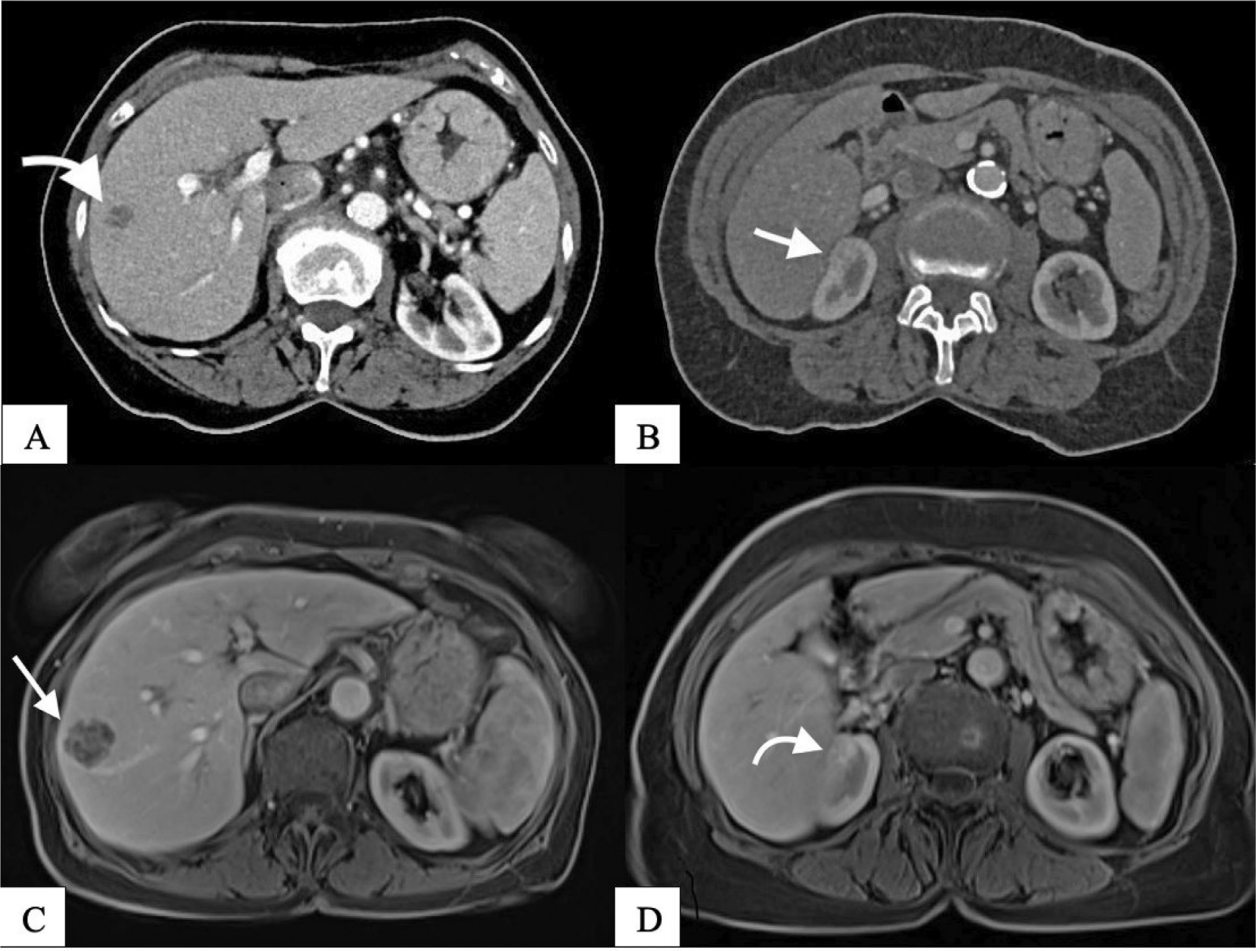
(A-D): Abdomen-CT and MRI 12 months after the initial diagnosis. 2A; upper left; axial view: slightly vascularized hypodense lesion on the segment VIII of the liver with a transversal 2, 4 cm. 2B; upper right; axial view: On the superior pole of the kidney a vascularized lesion with a 1, 6 cm. 2C; lower left; axial view: there is a pathological enhancement on the solid subcapsular mass. 2D; lower right; axial view: hypervascular lesion on the superior pole of the kidney with ca 1, 7 cm.
